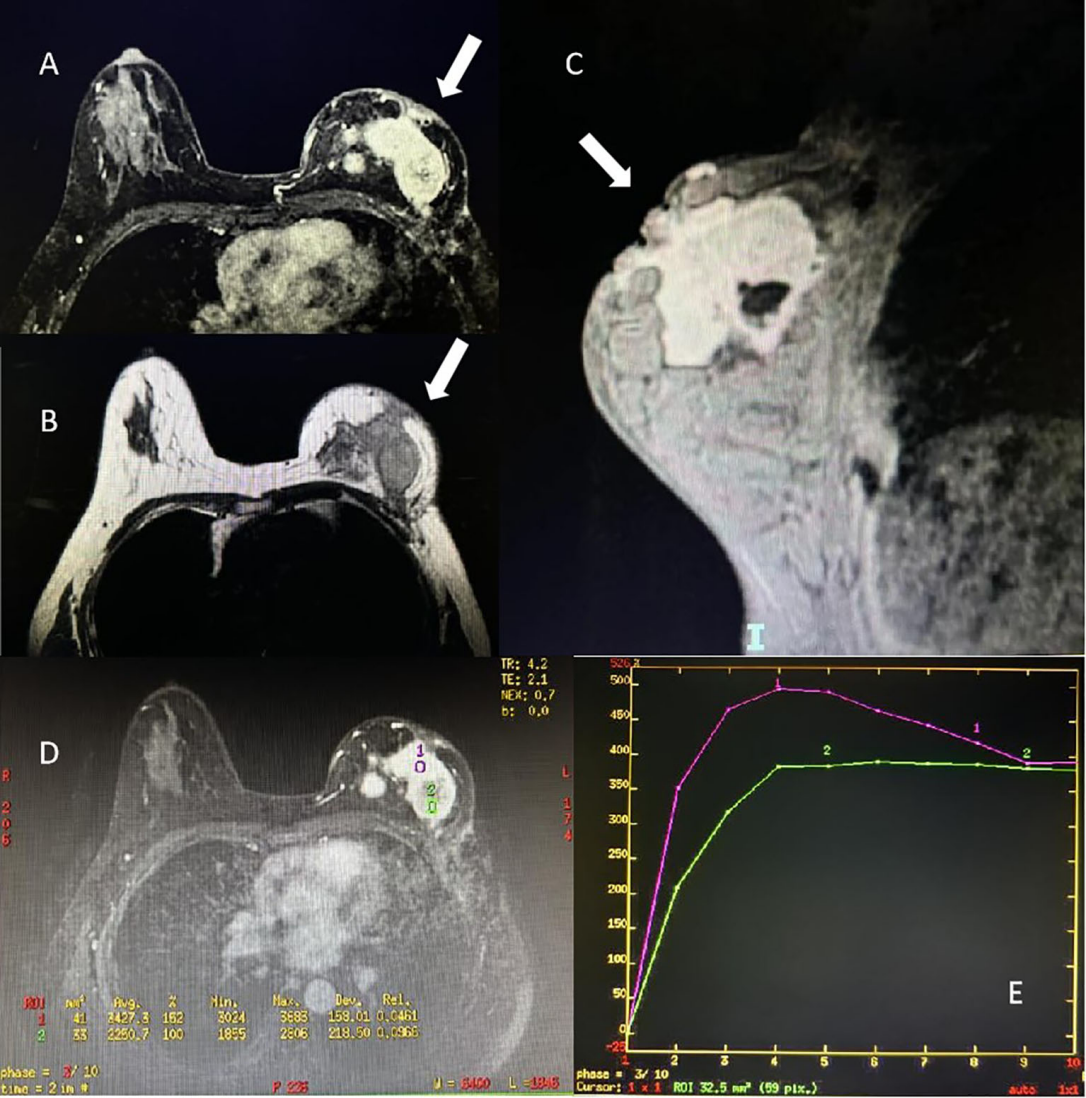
Magnetic resonance imaging of the patient of breast desmoid-type fibromatosis (white arrow). (A) Axial view of contrast enhancement of T1 signal. (B) Axial view of T2 signal. (C) Sagittal view of the mass of right breast. (D, E) Time-signal intensity curve of the pattern of contrast enhancement in region-of-interest.
